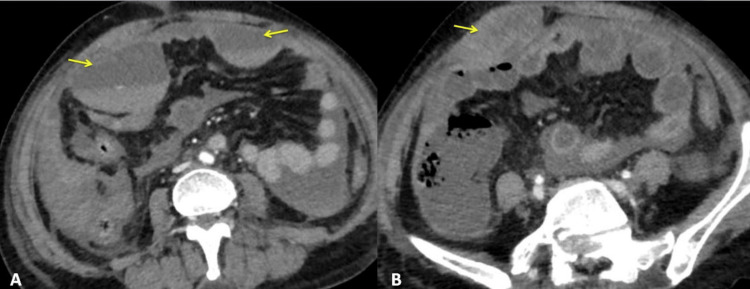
Computed tomography image (axial view) showing. A: Two well-defined hematomas in the right and left supra-umbilical region abutting the parietal peritoneum (arrows). B: The right rectus abdominis muscle appears bulky with an ill-defined hypodense rectus sheath hematoma (arrow).
